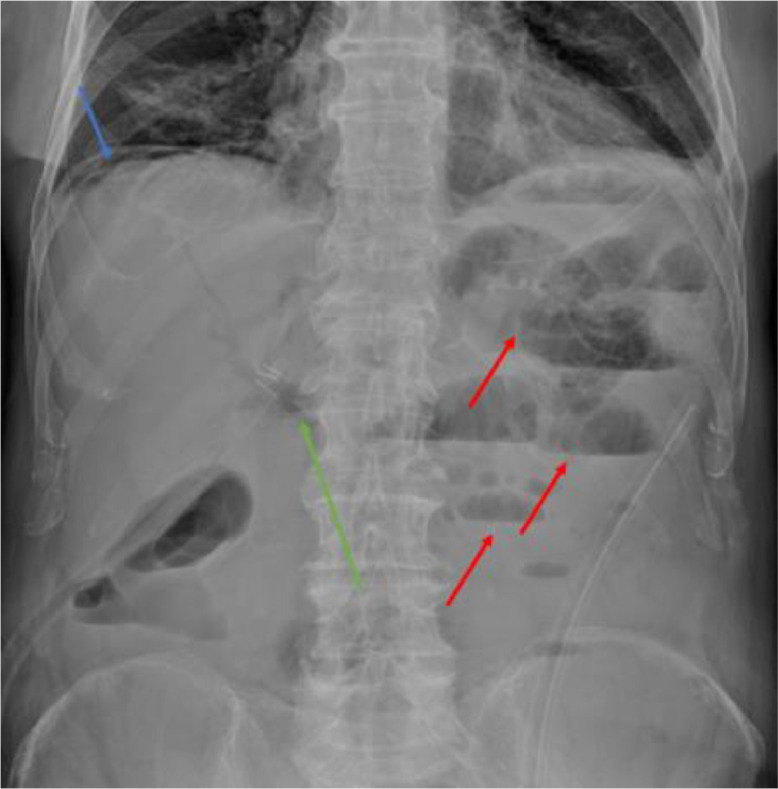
Abdominal X-ray performed 24 hours after surgical treatment for suspicious mechanical ileus showing subdiaphragmatic free air (blue arrow), a ladder-like pattern of fluid levels (red arrows), radiolucency corresponding to the biliary tree (green arrow).
